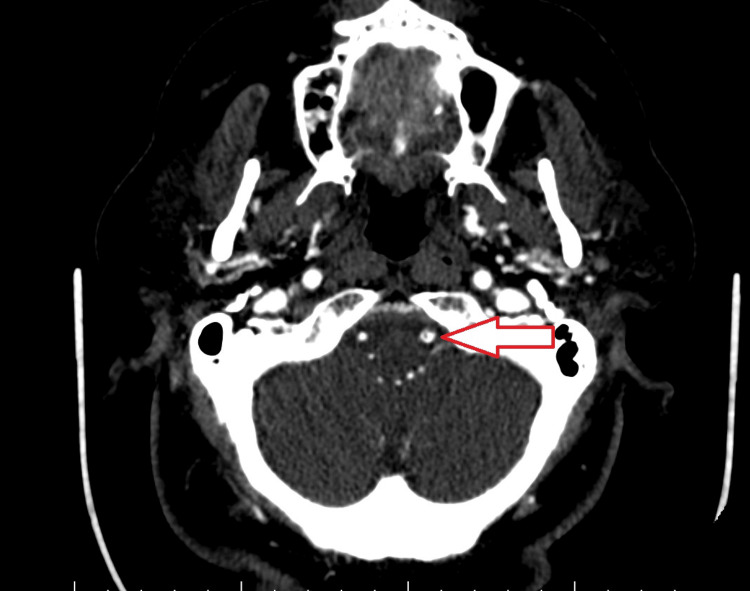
CT Angiography Showing Suspicious V4 Vertebral Artery Finding Computed tomography angiography (CTA) showing a thin hypodense flap in the V4 segment of the vertebral artery (arrow), raising suspicion for arterial dissection. Subsequent imaging did not confirm dissection.
